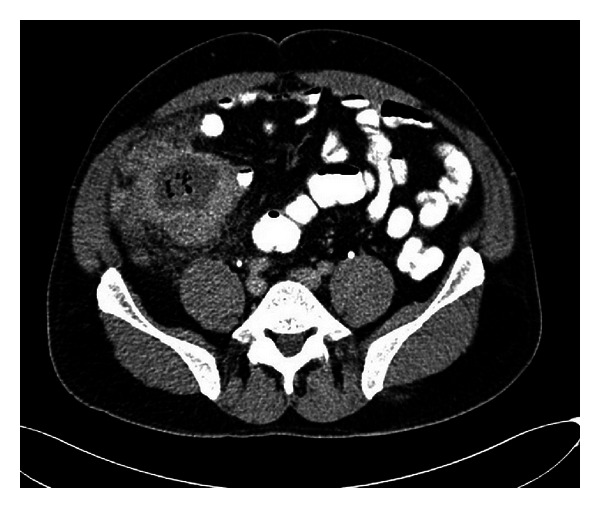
CT image of the mass located in the cecum.
